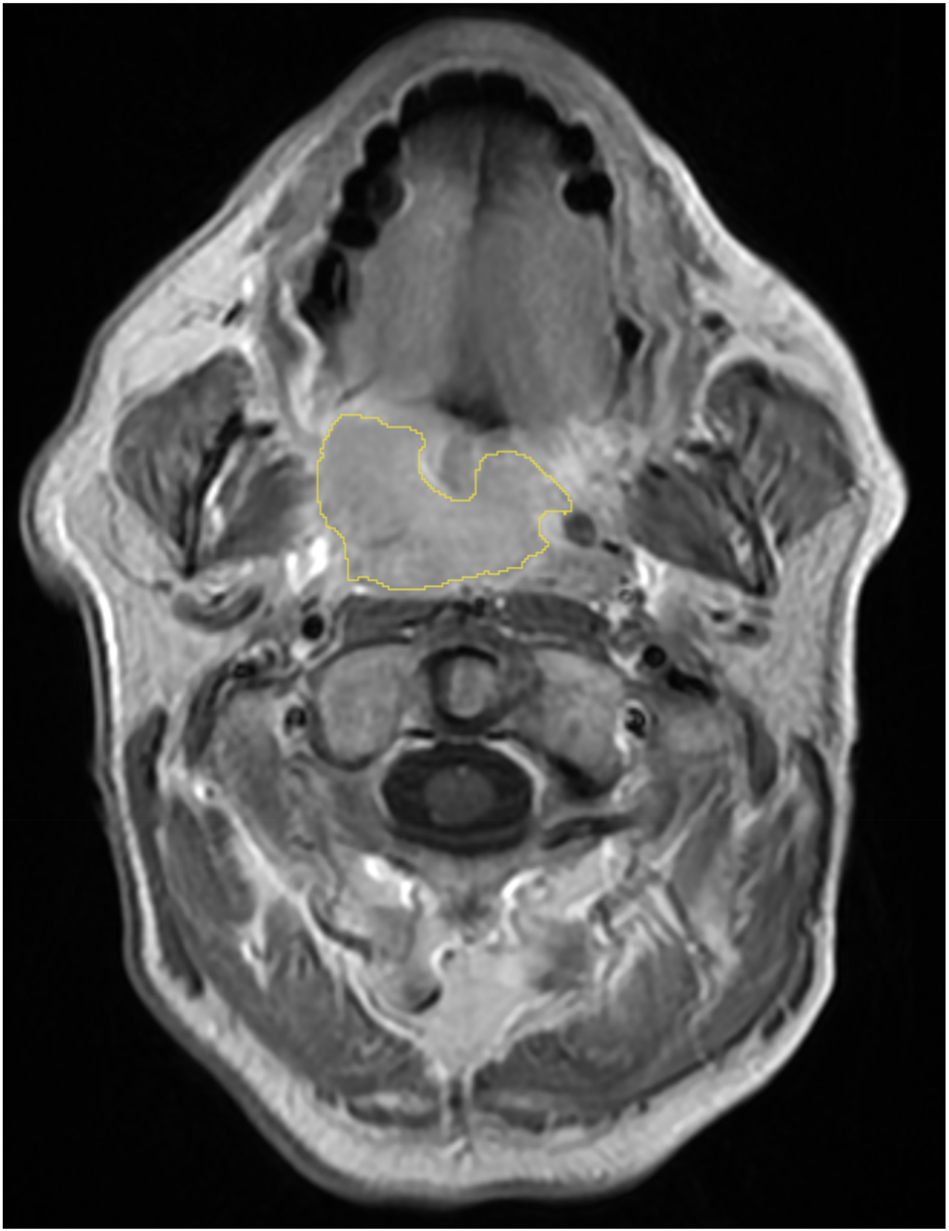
Delineation of primary oropharyngeal carcinoma on contrast-enhanced T1-weighted MRI. This figure demonstrates the segmentation strategy for viable tumour tissue (yellow ROI). Peritumoural inflammation was found with higher intensity surrounding the tumour tissue. Additionally, a necrotic mass was seen with low intensity anterior to the tumour tissue. Both peritumoural inflammation and necrotic mass were excluded by segmentation from viable tumour tissue
